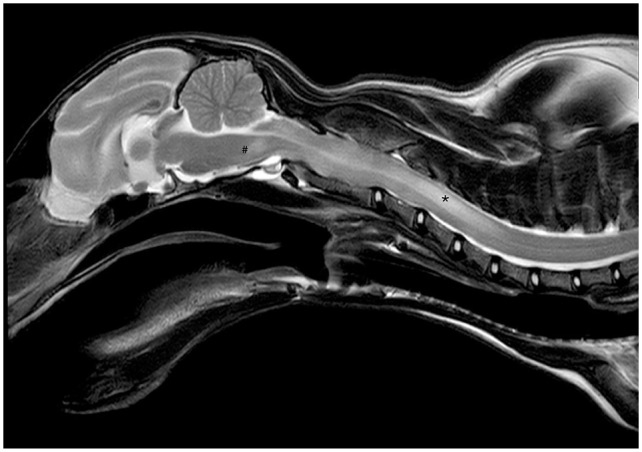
Sagittal T2-weighted (T2W) image of the brain and cervical spine. There was moderate spinal cord swelling and an extensive, asymmetrical, T2W and T1-weighted hyperintense lesion in the mesencephalon (#) extending along the cervical spinal cord until C5. The lesion is most pronounced at the level of C4 (*)
